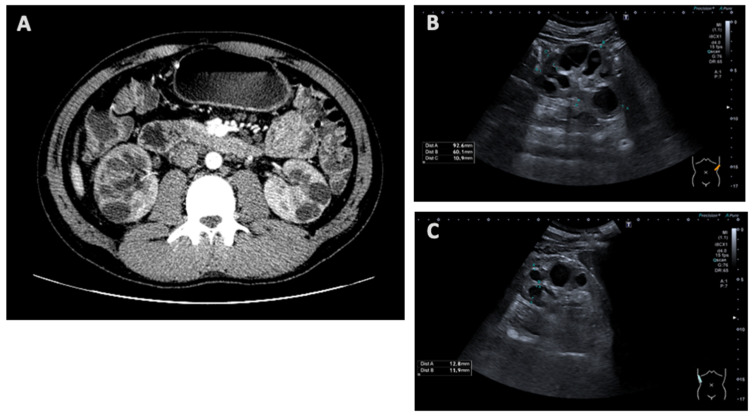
Abdominal computed tomography scan (A) and renal ultrasound scan (B,C) showing cystic kidneys in Patient 1.
